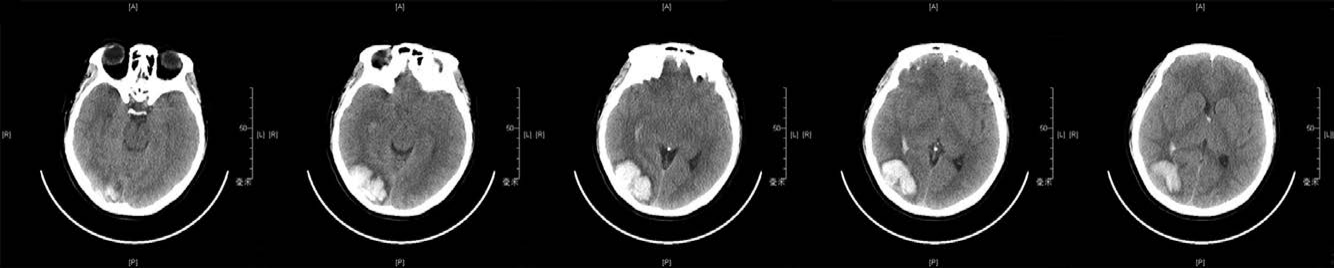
The preoperative CT images of the patient’s brain, showing the patient’s right occipital lobe hemorrhage and hematoma formation. CT = computed tomography.
